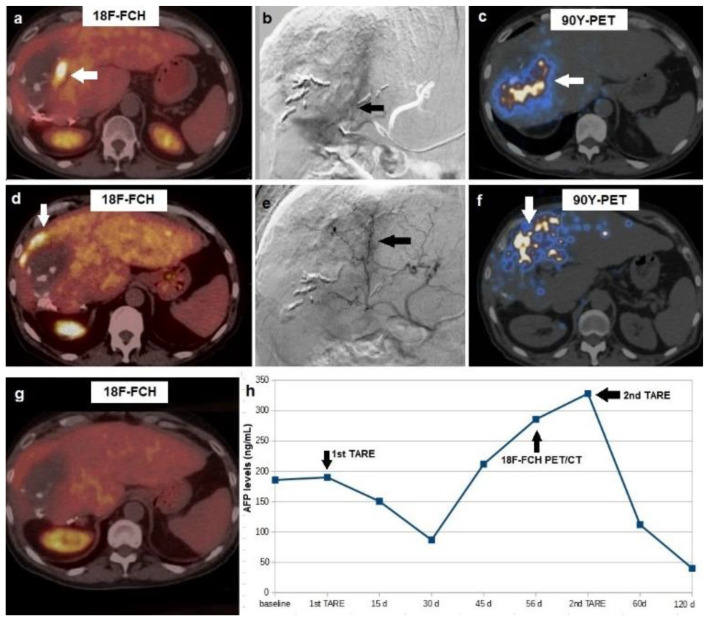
A 57-year-old male, affected by HCV-related cirrhosis and HCC in the right hepatic lobe, previously treated with laser ablation and surgical resection, referred to our center for ^90^Y-TARE. (a) Pre-treatment ^18^F-choline PET/CT demonstrated increased tracer incorporation in the infero-medial portion of the HCC lesion (arrow), which showed a central necrotic, hypodense area at co-registered CT slice. (b) Pre-TARE angiogram, performed after selective catheterization of the arterial feeder of the active tumor tissue detected by PET/CT, showed the hypervascularized tumor (arrow). (c) ^90^Y-PET acquired 4 h after TARE demonstrated ^90^Y-microsphere deposition in the treated tumor (arrow). (d) At 8-weeks post TARE PET/CT, a new-onset metabolically active HCC tissue was detected in the superior rim of the lesion (arrow), in spite of complete response of the tumor tissue previously located in HCC’s infero-medial portion. Therapeutic decision was to carry out a second ^90^Y-TARE: (e) a new angiogram was acquired after selective catheterization of the arterial branch for the upper hepatic segments (arrow). (f) ^90^Y-PET at 4 h post TARE demonstrated ^90^Y-microsphere accumulation in the superior portion of the HCC lesion. (g) Post-treatment PET/CT at 8 weeks after the second ^90^Y-TARE showed complete metabolic response. (h) Graph representation of alphafetoprotein trend during the different phases of the patient’s clinical management. Overall survival was 25 months.
